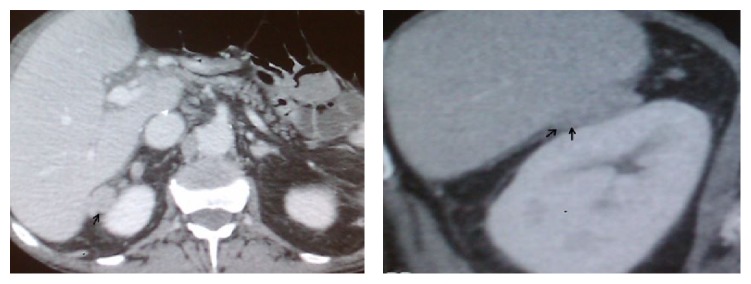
CT with intravenous contrast. Extrahepatic lesion between liver segment 6 and right kidney, largest diameter 2 cm.
